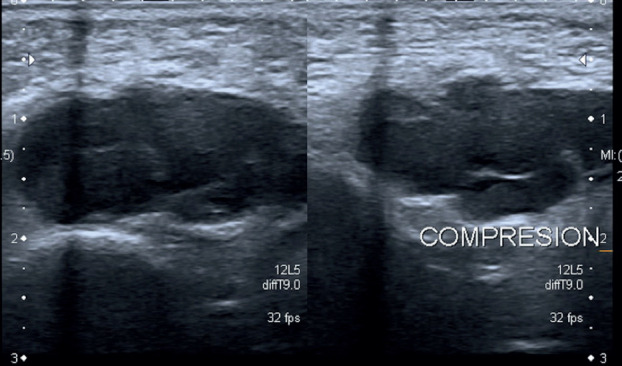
Ultrasound images. Lesion before and after compression; Lesion on doppler Mode. Echography of the left cheek: polilobulated hypoechogenic lesion, well defined and embedded in deep layer of subcutaneous tissue anterior to masticatory muscles without infiltration of approximately 1x2x3 cm. It is compressible. On its lateral left side, there are two small vessels with phasic flow suggestive of veins and it does not present flow on Doppler Mode. Taking into account the clinical and radiologic correlate, the lesion is suggestive of a thrombosed venous malformation.
